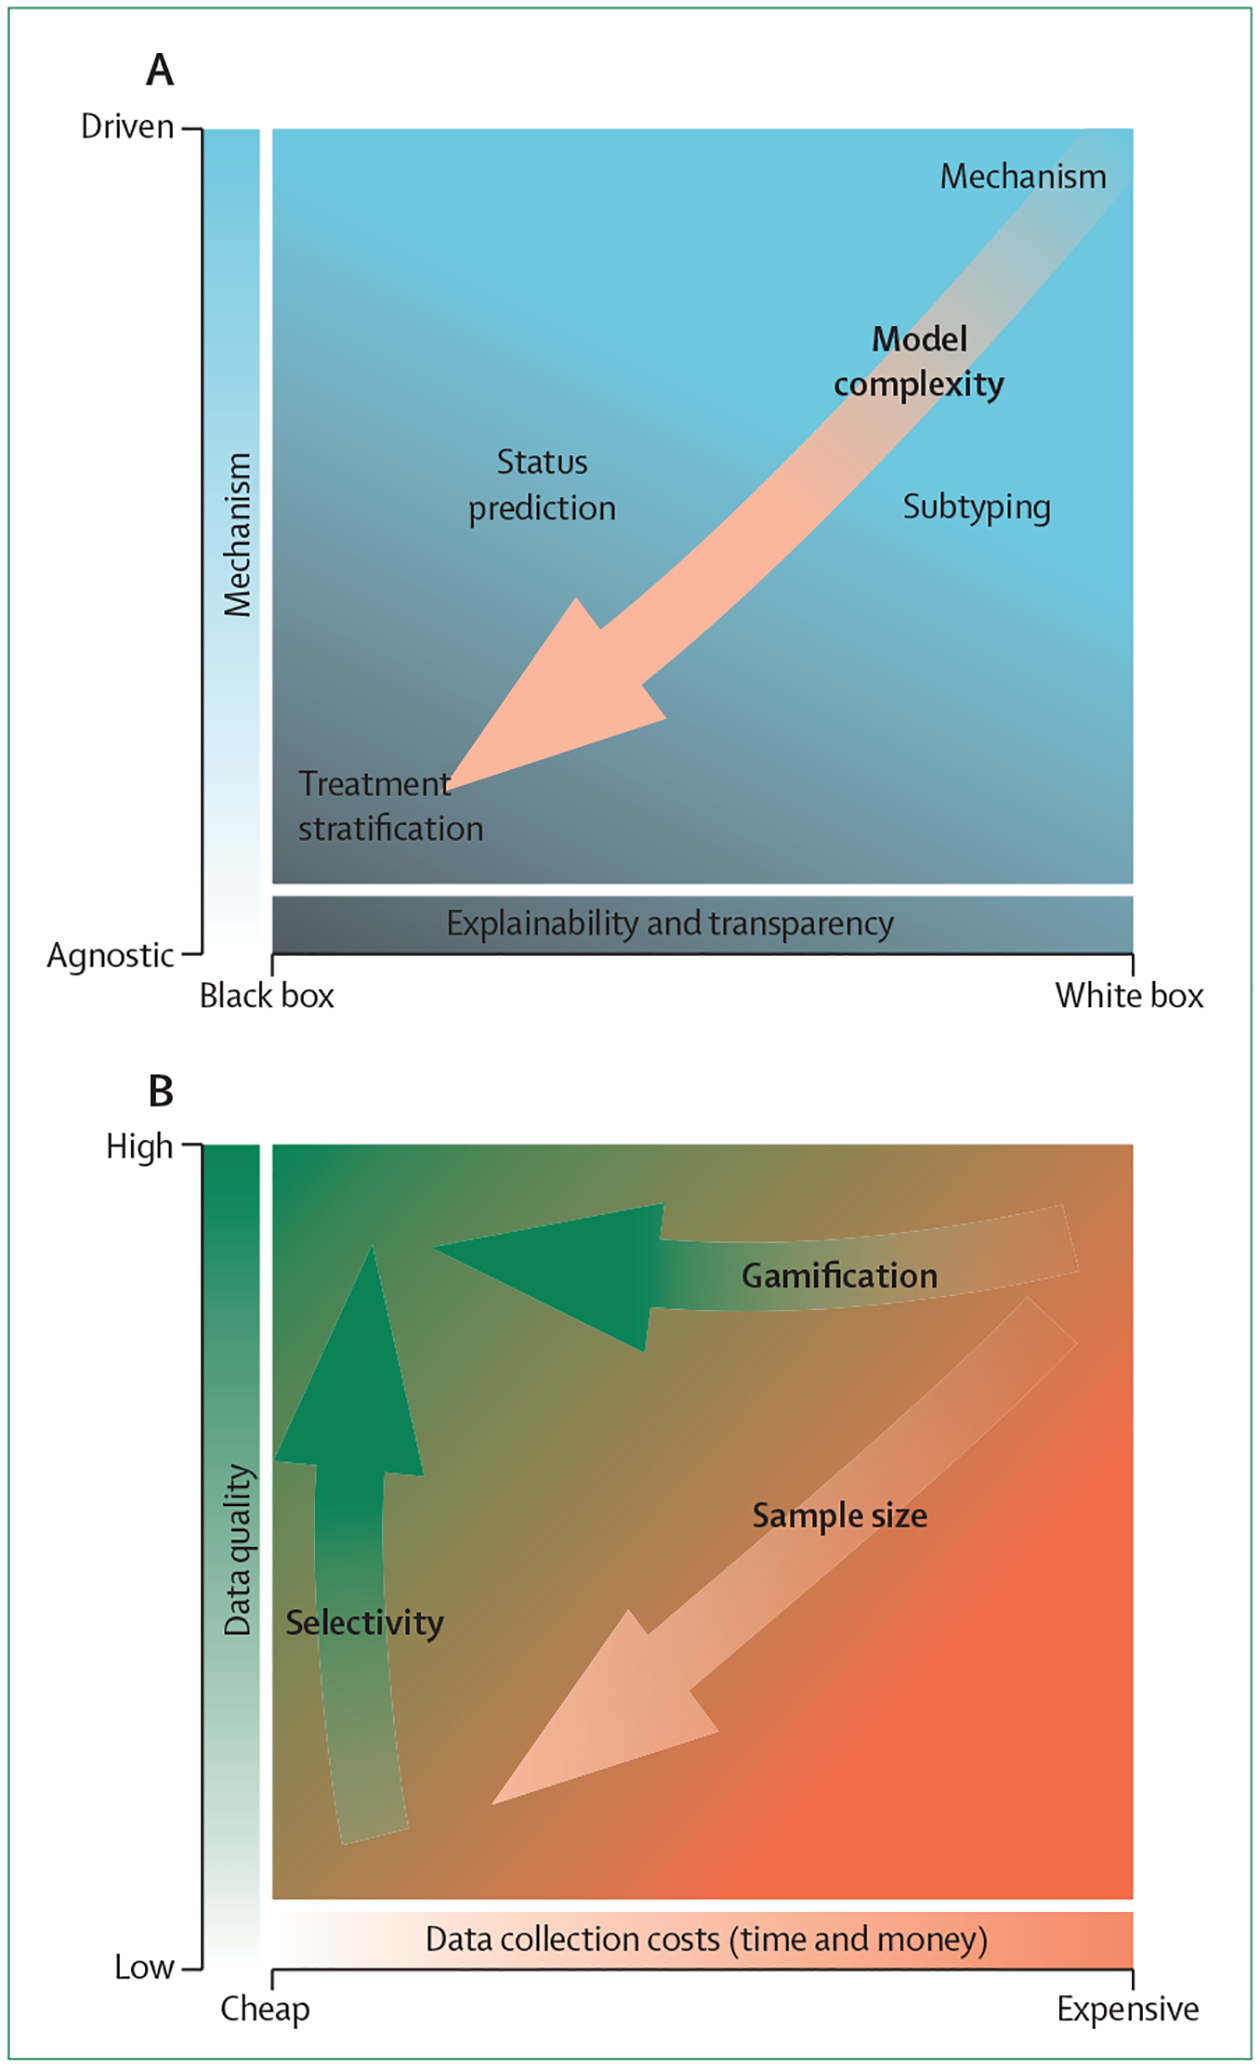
Trade-offs between models and data sources (A) Models differ in their transparency of the mechanisms, which determines their best use. Although most complex models often achieve higher predictive performance, white box models allow an understanding of the underlying mechanisms. (B) The choice of data source matters. High quality data (such as laboratory experimental studies) are often expensive (eg, functional MRI). Passive data collection is inexpensive, but the features are often unclear and not well defined. By transforming laboratory-based methods (eg, using gamification), substantially larger datasets can be collected at lower costs.
